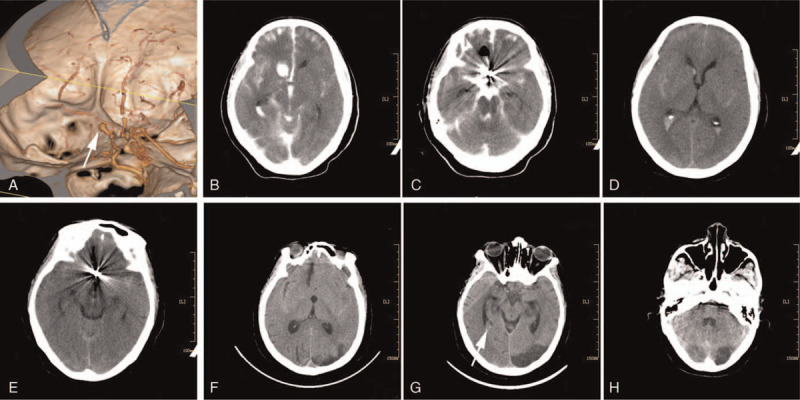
(A) Computed tomographic angiography (CTA) showed an anterior communicating artery aneurysm measuring 3 mm × 5 mm (as indicated by the arrow). (B and C) An urgent computed tomography (CT) after intraoperative bleeding showed subarachnoid hemorrhage (SAH) and intraventricular hemorrhage (IVH) mixed with the leaked contrast agent. (D and E) CT performed on day 6 showed hemorrhage in subarachnoid space, right frontal horn and bilateral occipital horns. (F–H) CT performed on day 12 showed a new delayed cerebral infarction in the left occipital lobe, a blood clot in the right temporal horn (as indicated by the arrow), and a reduction of SAH. No hydrocephalus or clots in the third ventricle (3 V) were noted.
